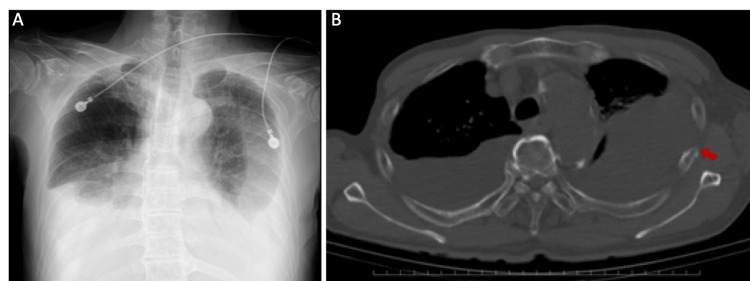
Imaging studies Chest x-ray shows bilateral pleural effusions (A). Computed tomography of the lung demonstrates a size discrepancy between the effusions and indicates a costal fracture (red arrow) (B).
